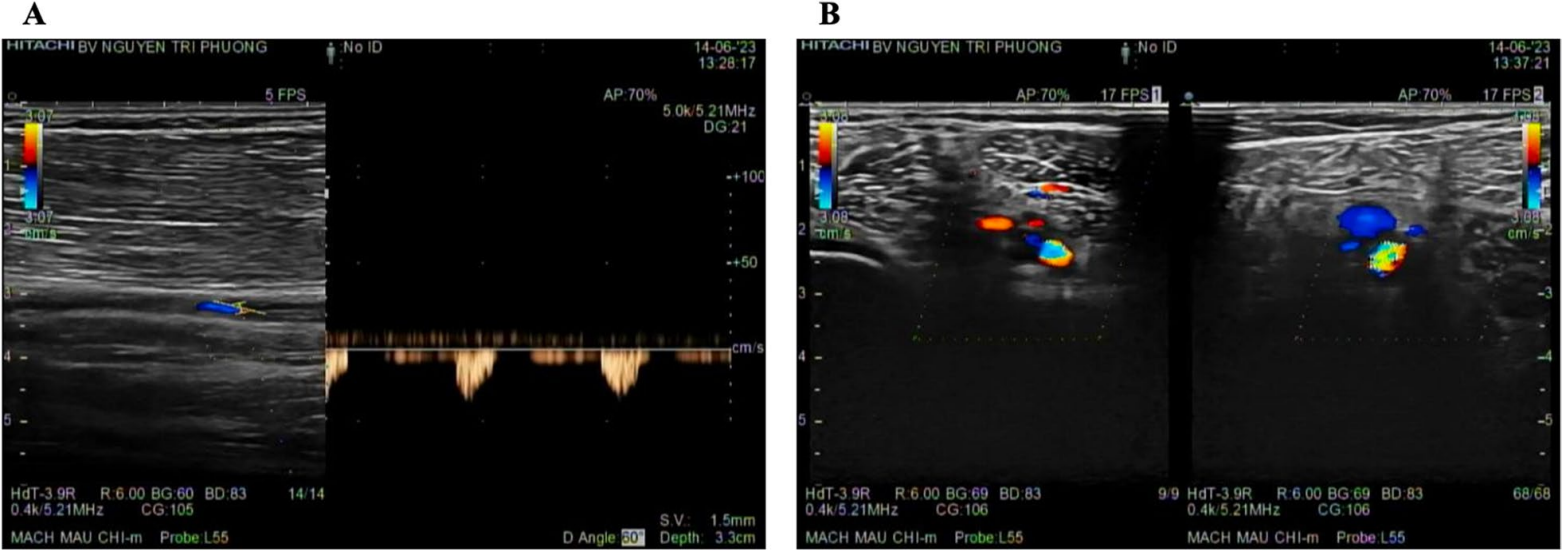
Doppler ultrasound of lower extremity’s vascular after 2 weeks of treatment. A Images of single-phase waveforms in the anterior and posterior tibialis arteries. B Thrombosis-induced partial occlusion of the tibialis arteries
